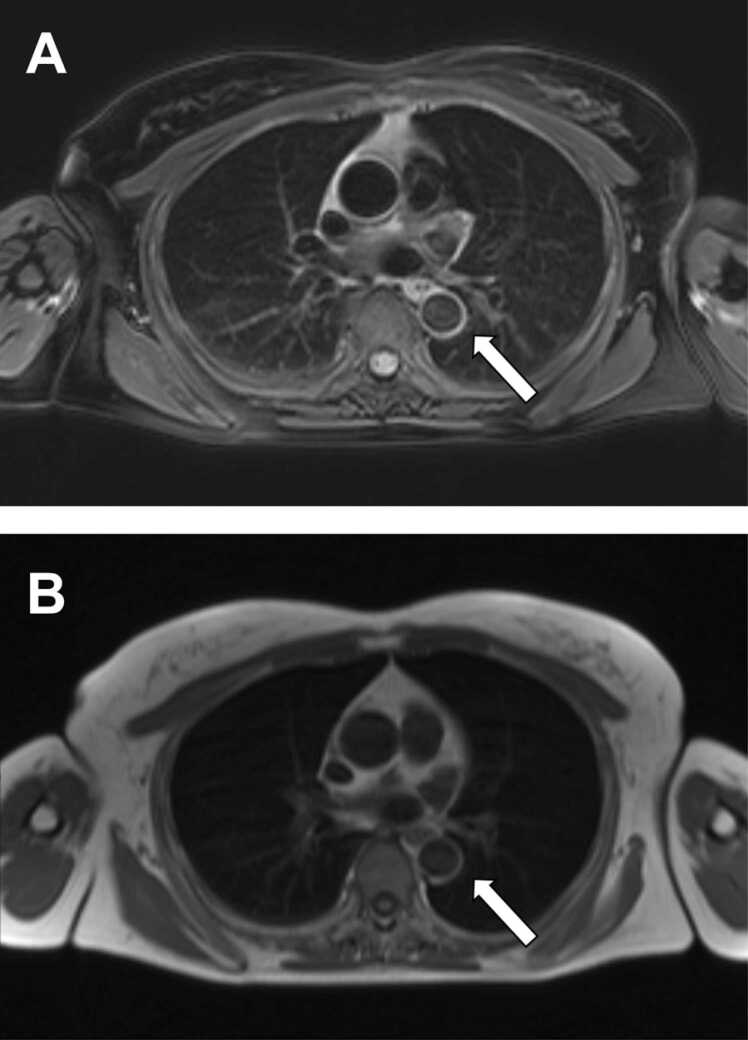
On MRI, vessel wall edema was observed in a total of 28 patients (21%). Correspondingly, axial non-contrast T2-weighted MR images *(A)* and T1-weighted fat-suppressed MR images *(B)* showed a hyperintense circular cuff around the thoracic aorta *(white arrows)*.
